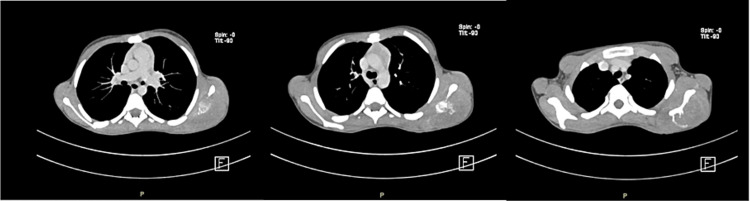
Computed tomography scan of the chest showing a large heterogenous destructive mass in the left scapula with calcified matrix, a large soft-tissue mass, and no evidence of lung metastasis
